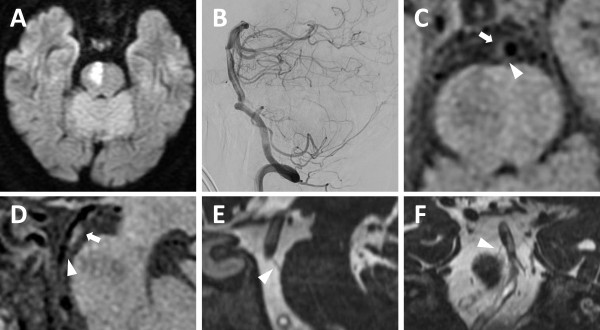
Magnetic resonance imaging. (A) Axial diffusion-weighted image showing an acute infarction in the territory of the right paramedian pontine artery that extends to the basal surface. (B) Sagittal digital subtraction angiography image showing moderate stenosis (<50%) in the basilar artery. Axial (C) and sagittal (D) non-contrast-enhanced three-dimensional fast spin-echo T1 imaging with variable flip angles (Cube T1) showing a plaque in the dorsal wall of the basilar artery (arrow). The orifice of the paramedian pontine artery is shown by the arrowhead. Sagittal (E) and coronal (F) three-dimensional fast imaging employing steady-state acquisition (3D-FIESTA) showing the right paramedian pontine artery (arrowhead). The ischemic lesion appears as a hypointense signal on the Cube T1 (C and D) and a hyperintense signal on the 3D-FIESTA (E).
